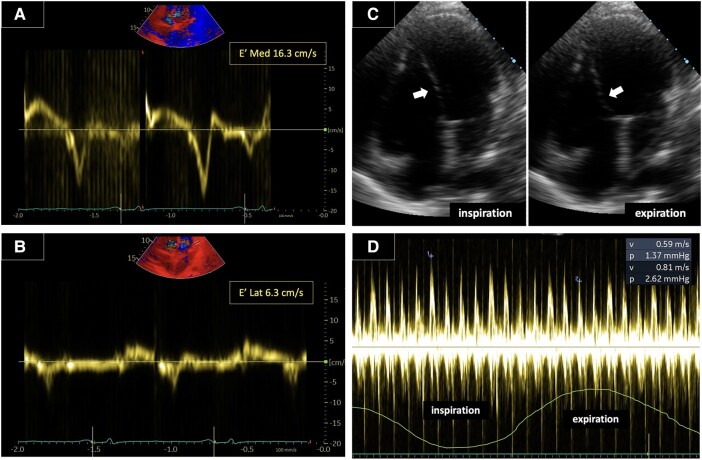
Echocardiogram during follow-up revealing signs indicative of constrictive pericarditis. (*A* and *B*) Tissue Doppler index (TDI) measurements demonstrating elevated E′ in the medial aspect and reduced E′ in the lateral aspect (annulus reversus). (*C*) Septal wall movement towards the left ventricle (LV) during inspiration and towards the right ventricle (RV) during the expiration phase, suggestive of further reduced LV filling during the inspiration phase (septal bounce/paradoxical septal movement). (*D*) Mitral inflow variation >25% with respiration, indicating significant fluctuations in mitral blood flow with breathing.
